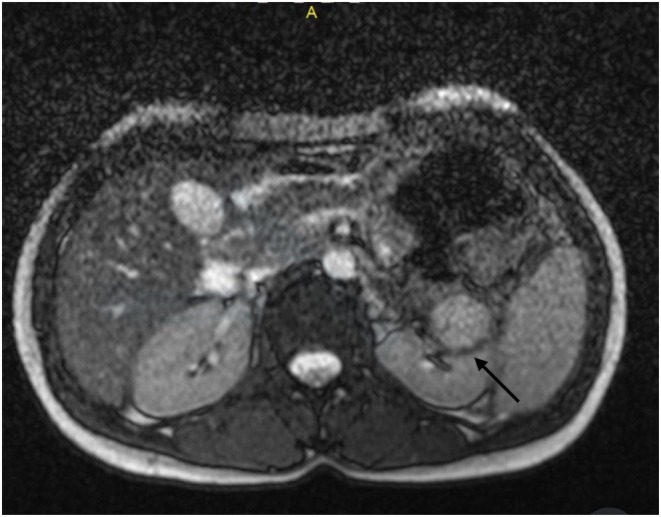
Axial T2‐weighted MRI image of the kidney post‐partial nephrectomy, performed following the occurrence of hematuria. The image reveals evidence of inflammation and hematoma formation.
